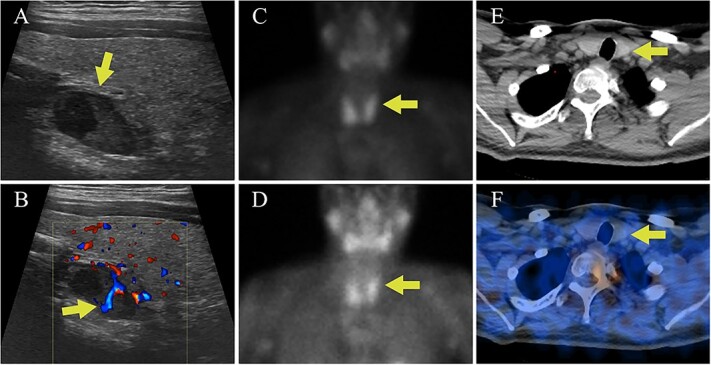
Case I parathyroid localization studies. (A) Thyroid US showed a heterogenous hypoechoic lesion posterior to the left thyroid lobe measuring 0.9 cm deep × 0.9 cm wide × 1.5 cm long (arrow, sagittal view). (B) Internal vascularity within the lesion was evident using doppler imaging (arrow, sagittal view). (C) On technetium-99m sestamibi scintigraphy physiologic uptake or the radiotracer was seen in the thyroid gland on the immediate images (arrow). (D) One hour delayed sestamibi images showed poor washout of radiotracer (arrow) but no areas of focal radiotracer retention. (E, F) No focal areas of uptake were observed on SPECT/CT images in the region correlating with the lesion identified on US (arrows).
